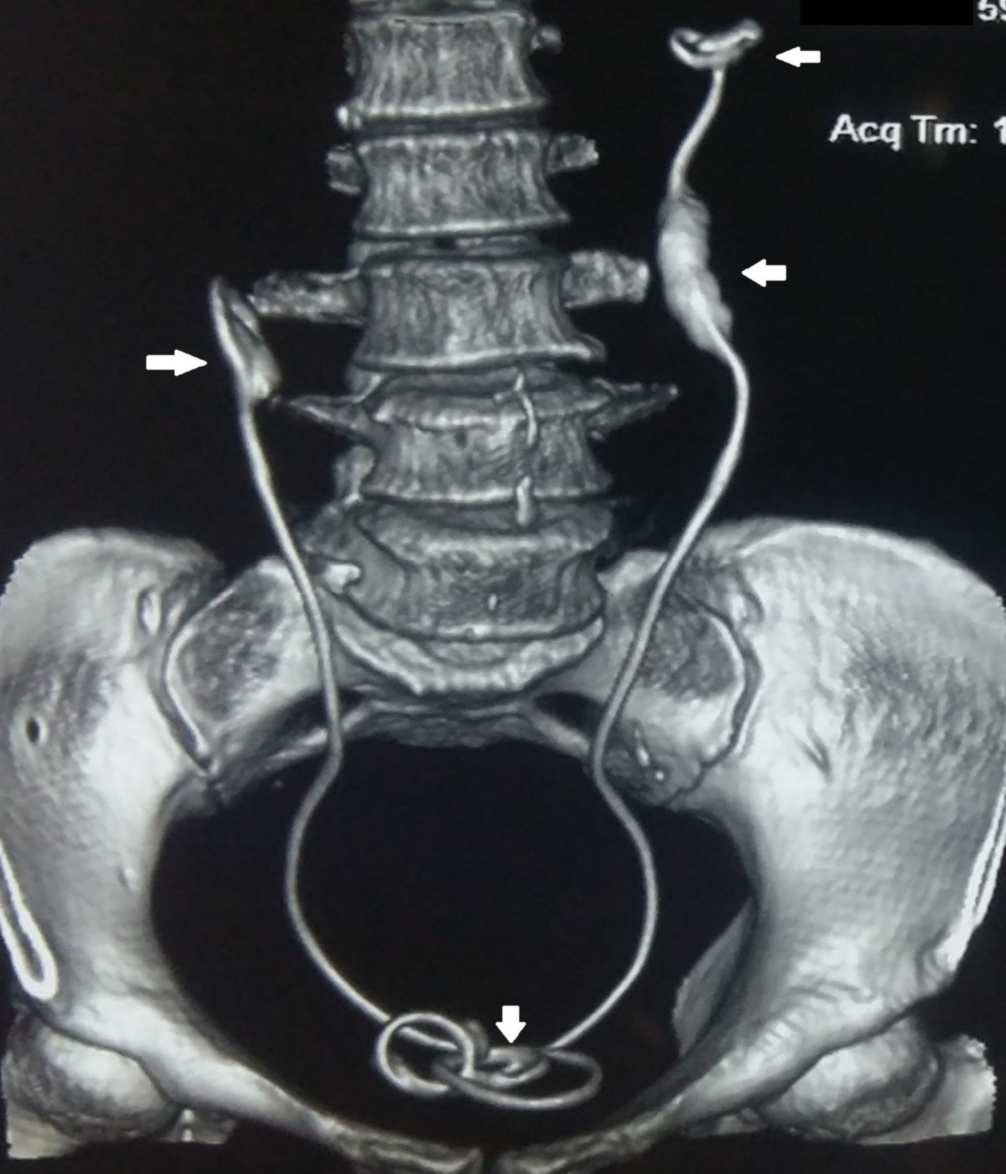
Reconstructed image of the noncontrast computed tomography depicting heavy encrustation of renal and vesical ends of both the stents (white arrows)
